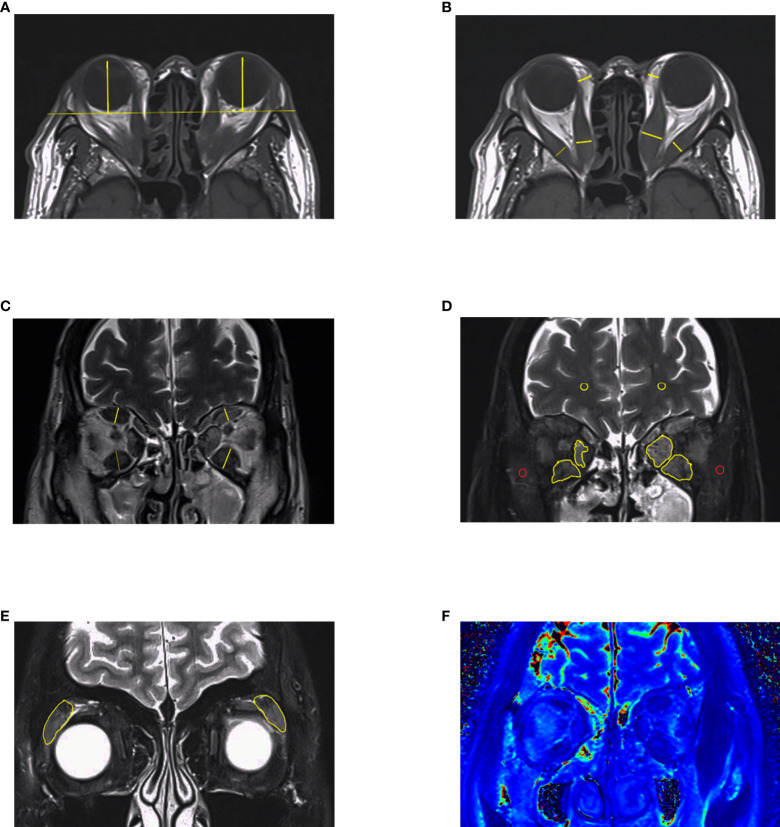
MRI measurements in GO. (A–C) Length parameters such as proptosis, thickness of extraocular muscles and thickness of orbital fat. It is noteworthy that thickness of medial rectus and lateral rectus muscles should be measured in axial images. (D, E) Signal intensity of extraocular muscles and lacrimal glands got from Dixon-T2WI sequence. The signal intensity of temporalis muscle (red circle) or white matter (yellow circle) on the same slice was used to calculated signal intensity ratio (SIR). (F) EOMs displayed on T2 mapping. This figure is original.
